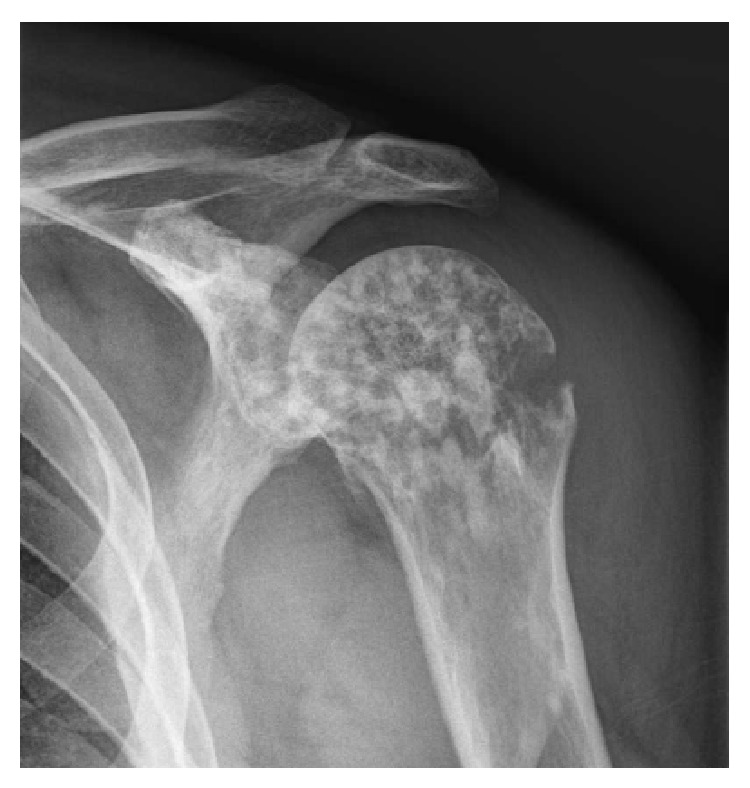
Radiograph of the left shoulder showing a metaphyseal proximal slightly displaced fracture of the humerus and a heterogeneous bone structure with epiphyseal and metaphyseal predominance.
